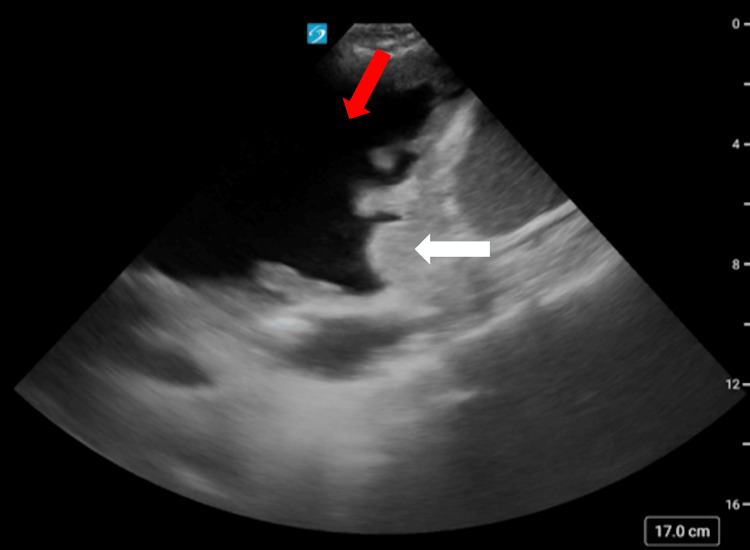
Point-of-care ultrasound. Point-of-care ultrasound of the left chest in the midaxillary line showing left-sided pleural effusion (red arrows) with pleural nodularity (white arrows).
